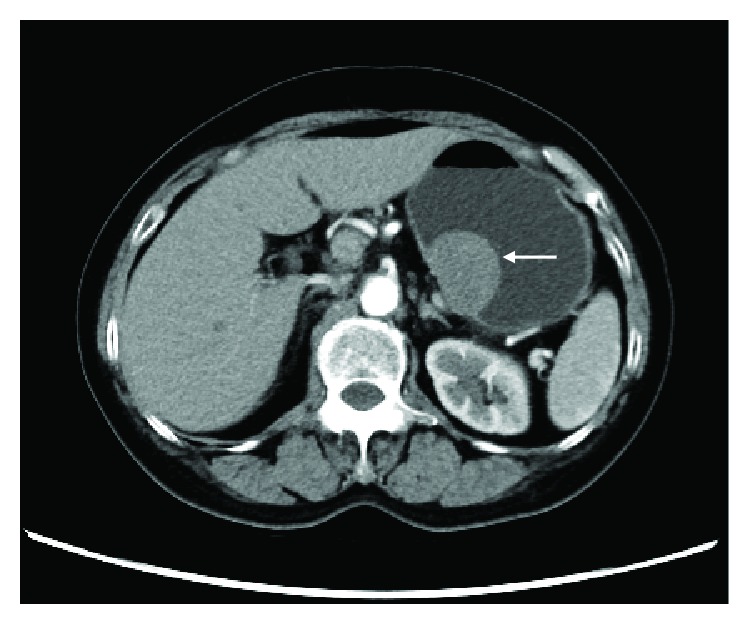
An approximately 3.9^∗^2.8 cm gastrointestinal tumor on the lesser curvature of the stomach body seen on enhanced CT imaging (white arrow).
